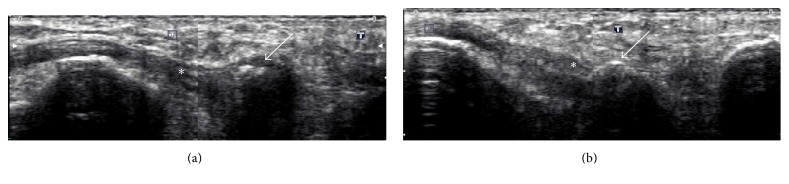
60-year-old female ultrasonography of the long axis of the peroneus longus tendon. (a) Right foot: complaint side demonstrating a thickened and heterogeneous peroneus longus tendon (asterisk) and irregular and fragmented os peroneum, associated with swelling of the surrounding soft tissues. (b) Left foot: contralateral side for comparison, demonstrating a preserved echotexture of the peroneus longus tendon (asterisk) and regular contours of the os peroneum without changes in the surrounding soft tissues.
